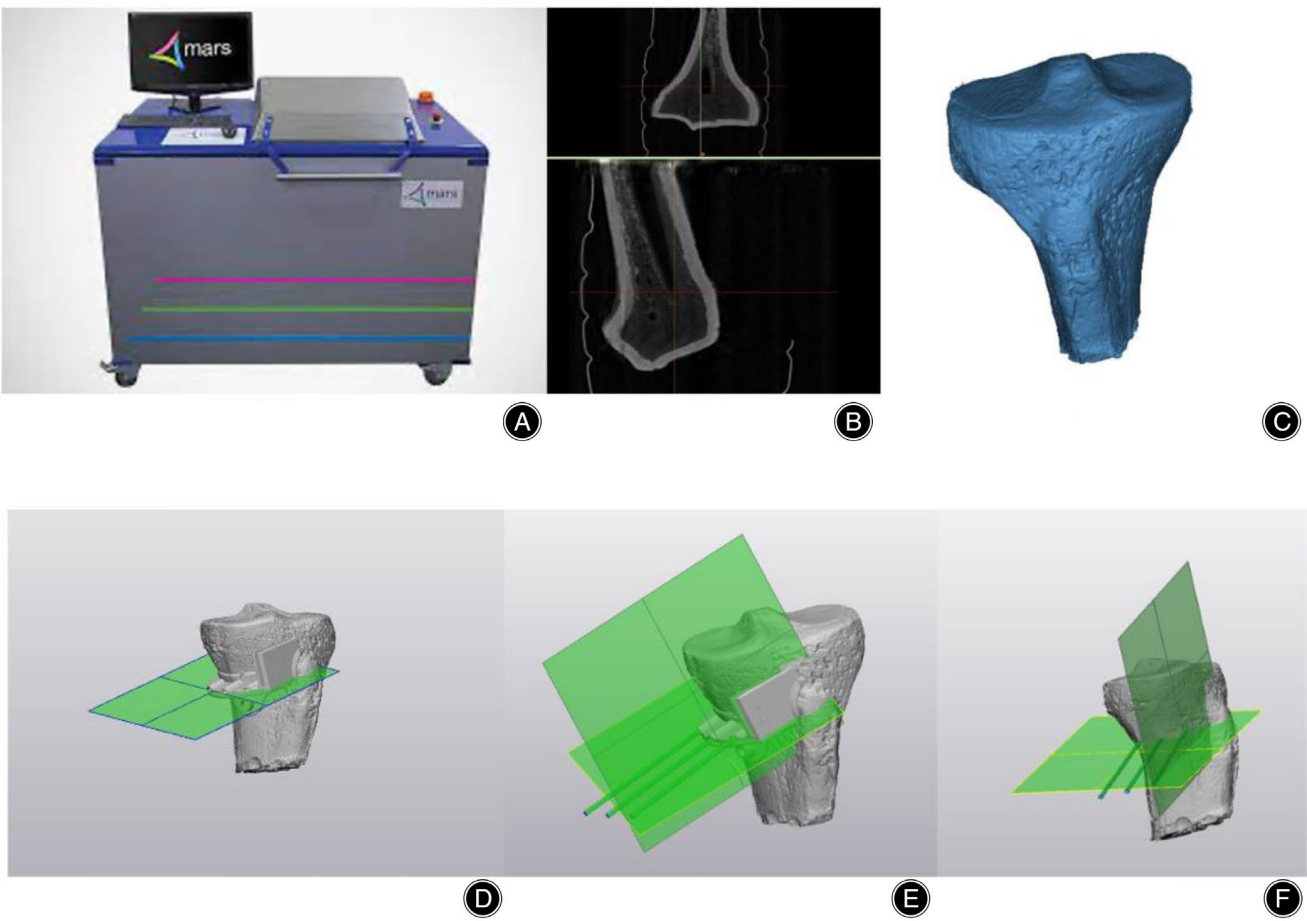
(A,B) The Mars CT scan machine and image in Mars CT, respectively. (C) The segmented 3D model. (D) The cutting‐plane planning. (E) Demonstrates the screw planning. (F) The final output of two cutting planes without the Jig.
